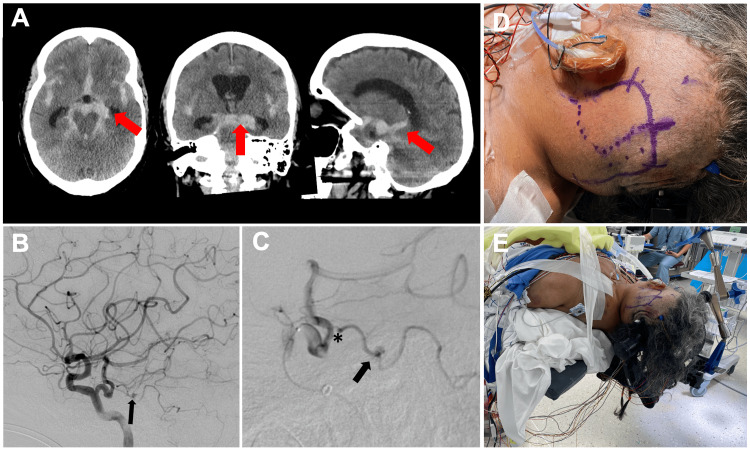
An 82-year-old female was found to have modified Fisher grade IV diffuse SAH on a head CT scan with IVH and obstructive hydrocephalus (A) Head CT scan in axial, coronal, and sagittal reconstruction indicating SAH located in the basal cisterns with IVH and communicating hydrocephalus (arrows). (B) Lateral left internal carotid artery angiogram showing a left Saltzman Type 1 PTA and a left AICA aneurysm (a2 segment) (arrow). (C) Selective PTA microcatheter injection showing the origin of AICA from PTA with origin stenosis (asterisk) and A2 aneurysm (arrow). (D) Hockey stick skin incision and marking the course of the left OA (dotted line) using navigation and Doppler for potential OA-AICA bypass. (E) Supine patient positioning with head turned for an extended retrosigmoid approach using a radiolucent head clamp for intraoperative angiogram. AICA, anterior inferior cerebellar artery; IVH, intraventricular hemorrhage; OA, occipital artery; PTA, persistent trigeminal artery; SAH, subarachnoid hemorrhage
